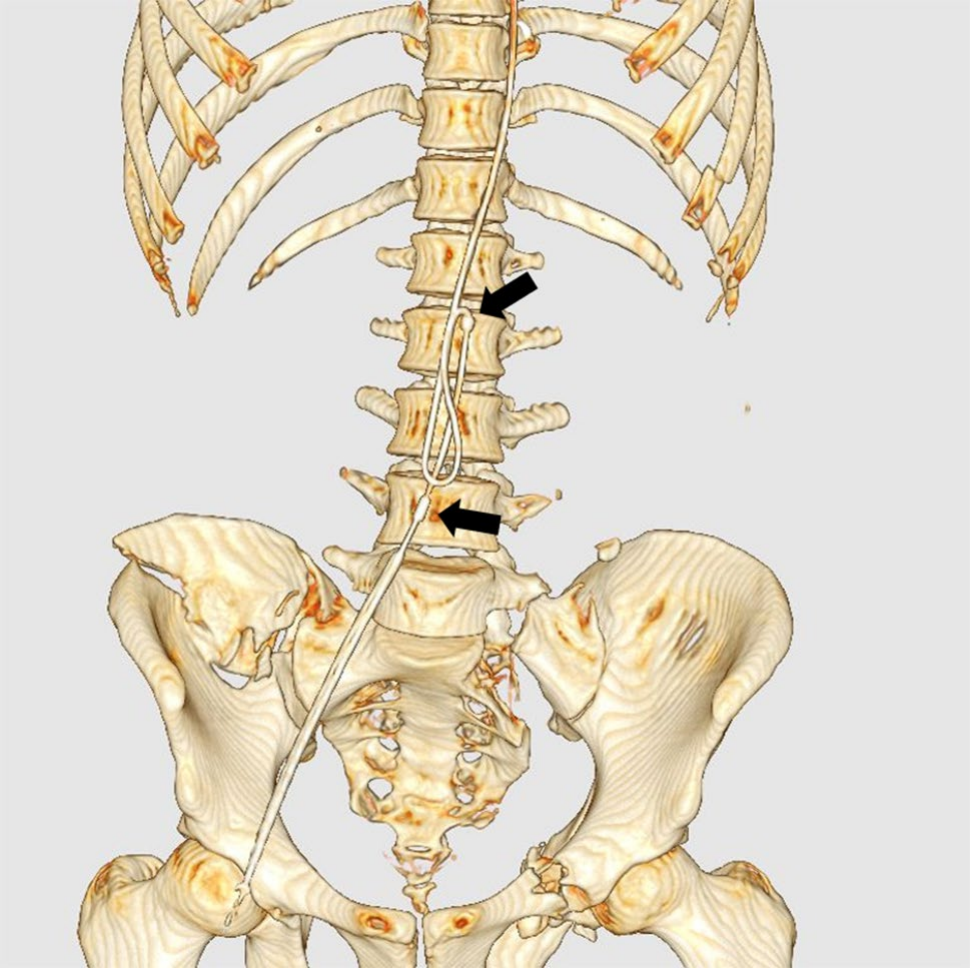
Three-dimensional computed tomography showing loop formation of the guidewire. The balloon catheter could not be inserted due to the looping of the guidewire. The black arrows indicate the radiopaque markers of the balloon catheter
